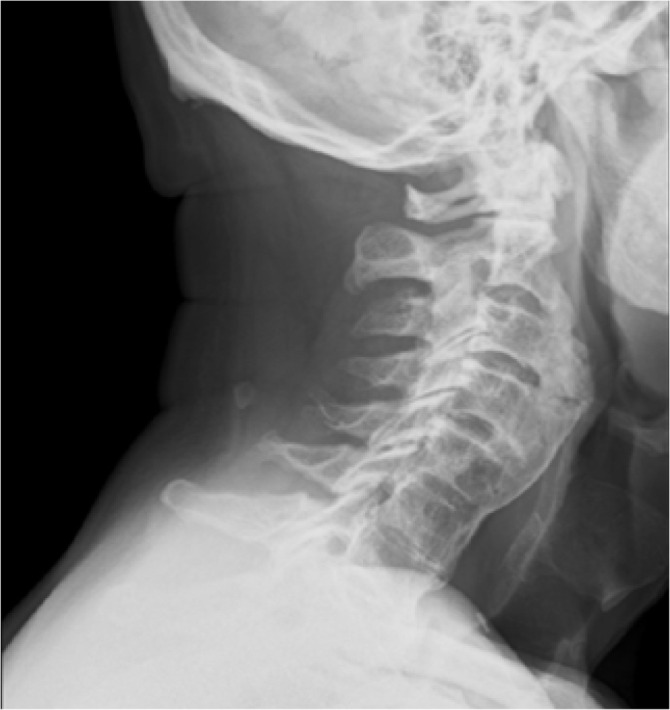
Lateral cervical spine x-ray showing anterior bone formation consistent with DISH with mass effect at the laryngopharynx.
